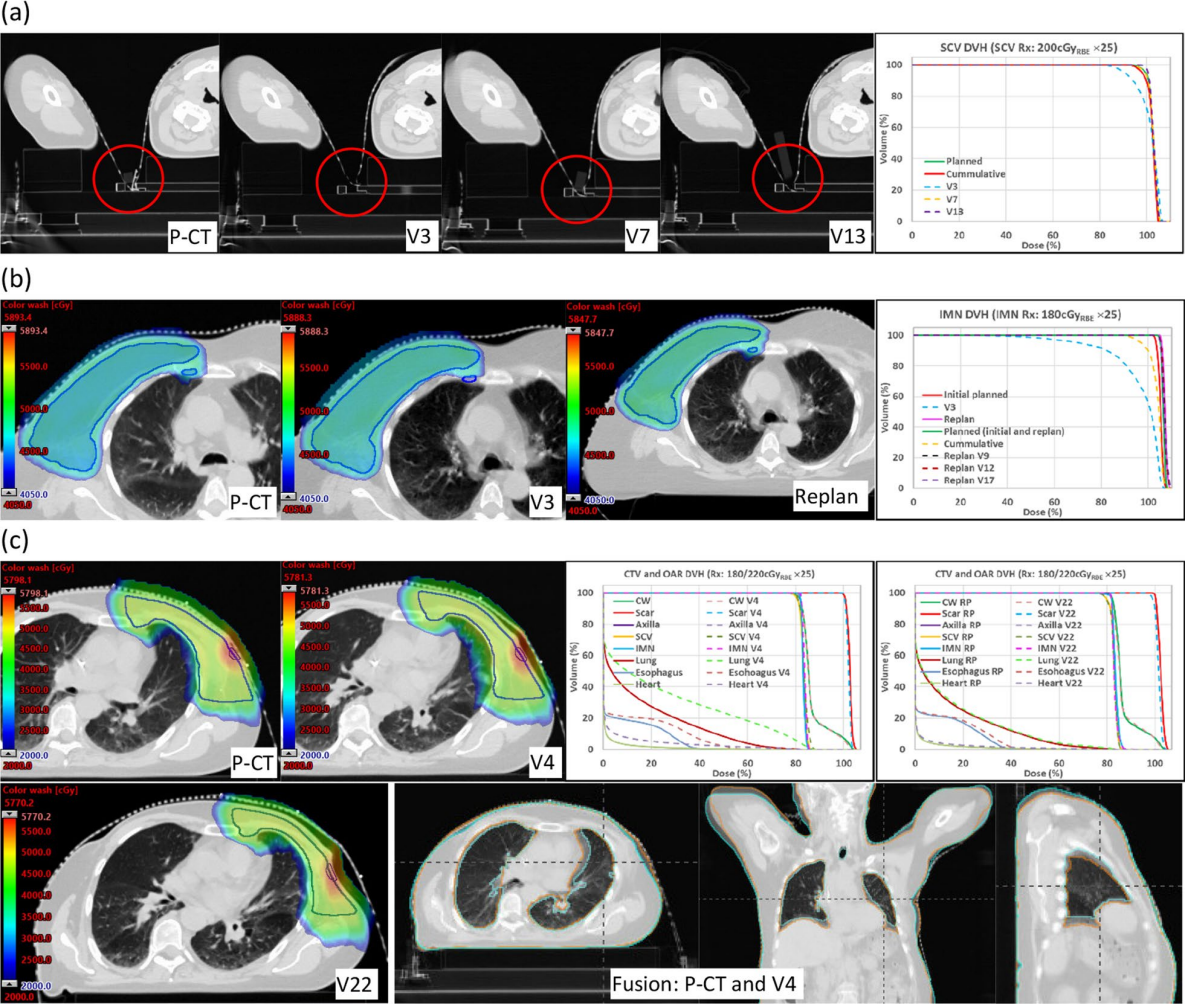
Masked patients with LDDs. a A patient with SCV LDD on V3 due to loose fixation point between upper arm and neck. SCV dose improved on V7 and V13 after the fixation point was secured. b The patient with IMN LDD on V3 due to inconsistent gap between mask and patient on P-CT and V-CT. Replan improved IMN dose for V9, V12, and V17. c A patient with increased gap between mask and skin on V-CTs compared to P-CT due to reduced CW tissue swelling, which caused lung and heart LDD. Replan improved lung and heart dose on V22. P-CT, planning computed tomography; V-CT, verification computed tomography; Vx, V-CT at fraction x; SCV, supraclavicular node; DVH, dose volume histogram; Rx: prescription; Gy_RBE_, Relative-Biological-Effectiveness dose in Gray; IMN, internal mammary nodes; CW, chest wall; CTV, clinical volume; OAR, organ at risk; RP, replan; LDD, large dose deterioration
